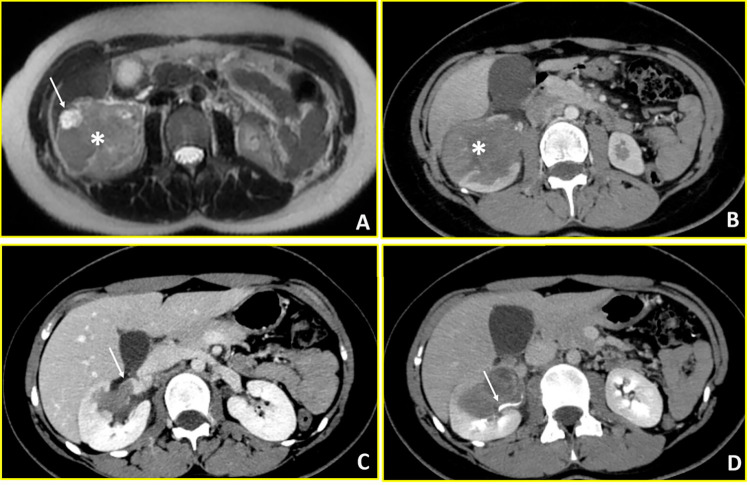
Ewing sarcoma (Case 3). Multimodality imaging demonstrates a large heterogeneous mass in the right kidney with vascular and collecting system involvement. (A) T2-weighted axial MR image reveals a heterogeneous renal mass (asterisk) with extensive intralesional necrosis appearing as areas of marked hyperintensity (arrow). (B) Axial arterial-phase contrast-enhanced CT image shows a predominantly hypovascular mass (asterisk). (C) Axial venous-phase image demonstrates tumor extension into the right renal vein (arrow). (D) Axial urographic-phase image shows infiltration of the renal collecting system with involvement of the renal calyces (arrow).
